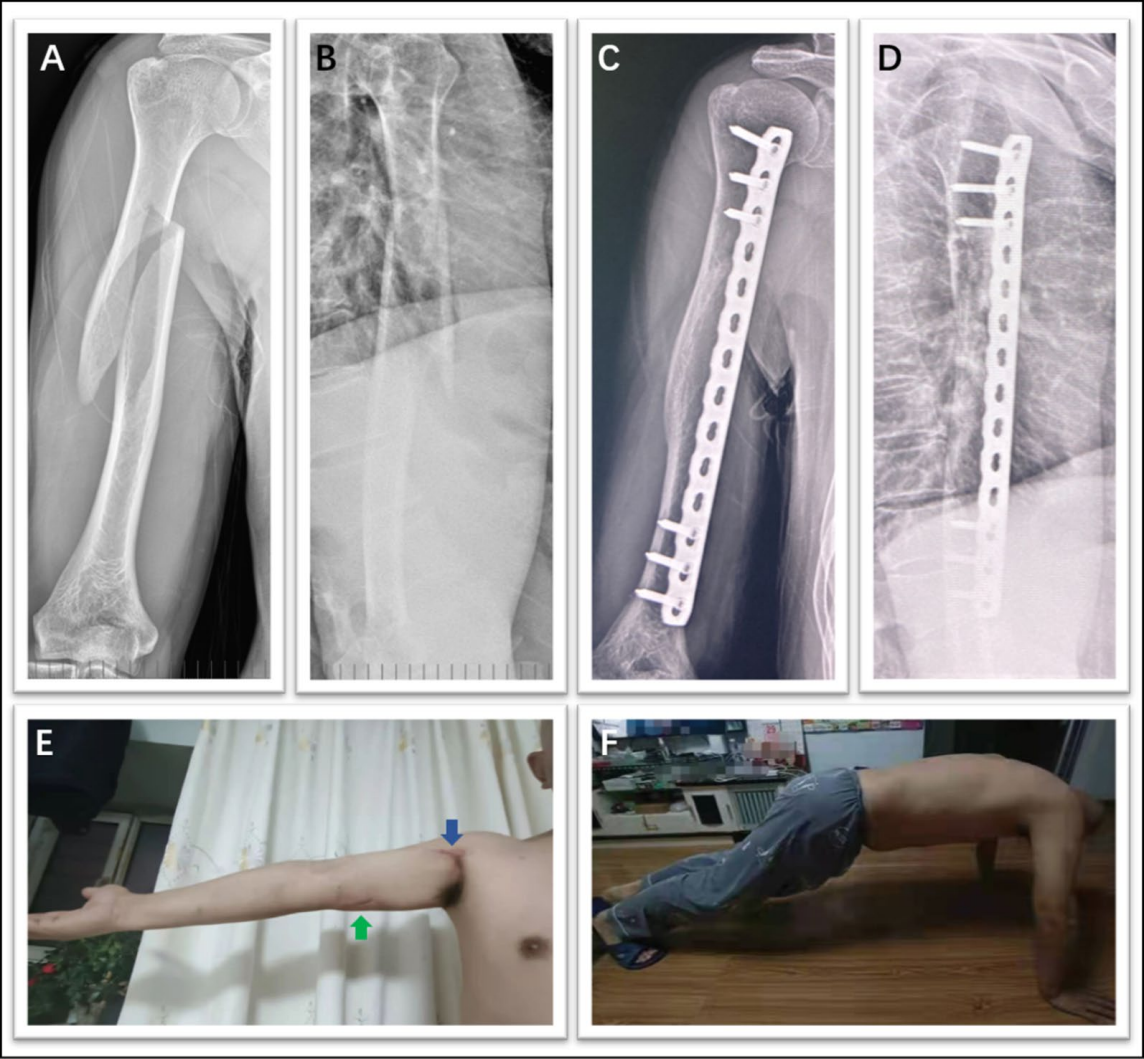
This figure shows a typical case. The patient fell while walking down stairs and sustained a middle fracture of the right humeral shaft. A–B Preoperative X-rays. C–D X-rays taken 3 months after surgery, with full recovery of function. E Proximal (*blue arrow*) and distal (*green arrow*) incisions. The incision is on the medial side, so the distal scar from the surgical incision is more hidden. F Three months after surgery, with full recovery of function
